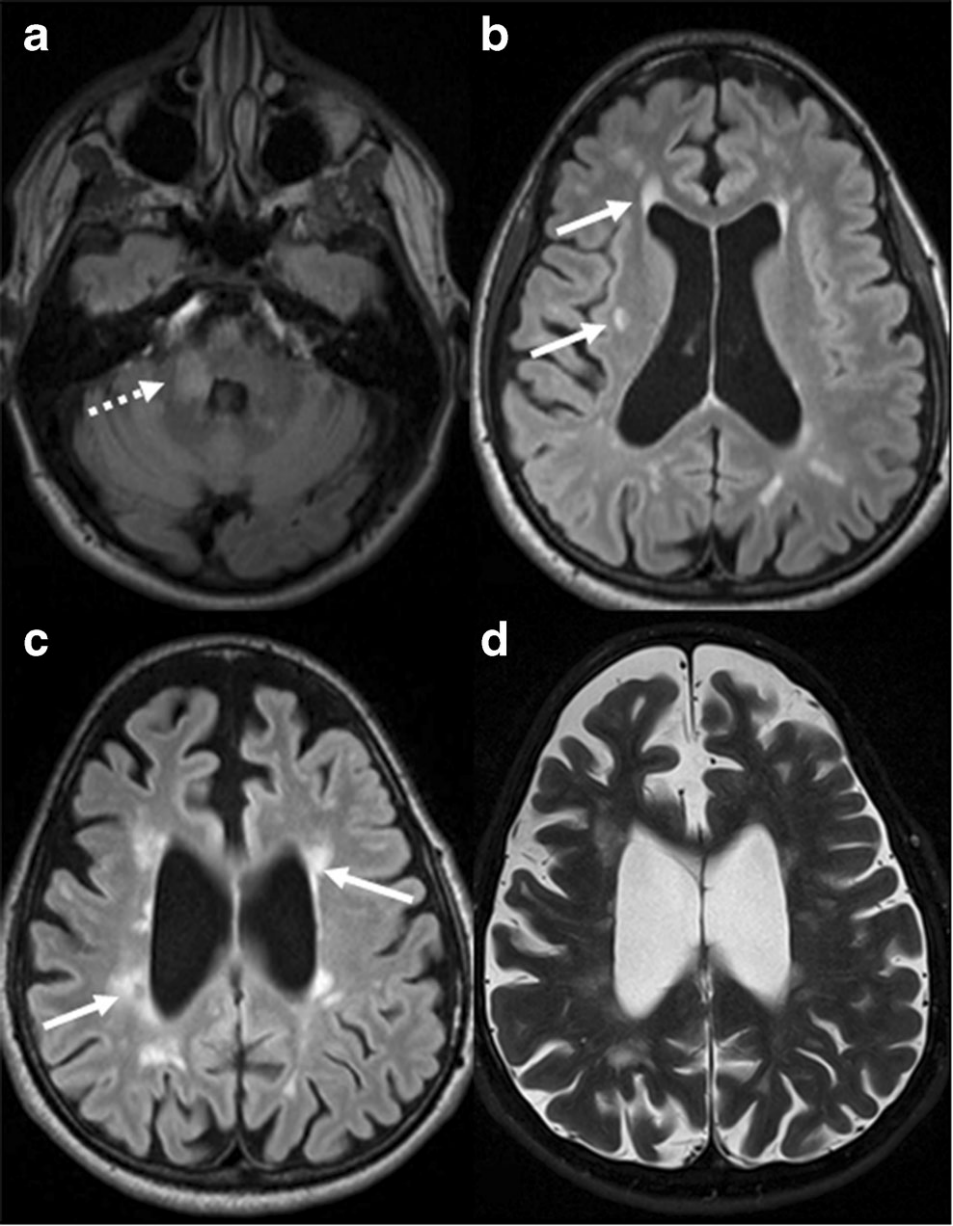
Mutliple Sclerosis (MS). A 40-year-old female with history of relapsing remitting multiple sclerosis (RRMS) for the last 5 years, recently developed progressive memory decline. a axial FLAIR image of the posterior fossa shows a hyperintense demyelinating lesion in the right brachium pontis (*white dashed arrow*). b, c & d axial FLAIR and T2W images at the level of lateral ventricles demonstrate multiple hyperintense lesions in periventricular white matter (*white arrows*) and generalized atrophy
